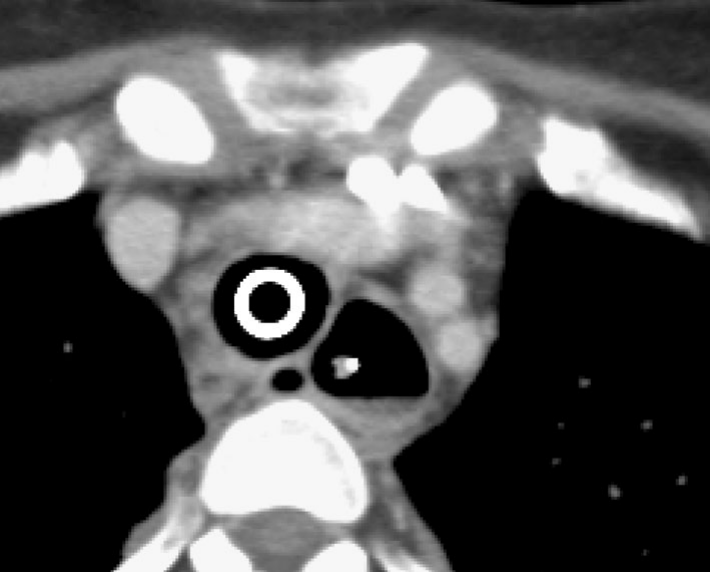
A follow-up computed tomography (CT) scan performed on the 15th day from the onset demonstrating the improvement in the diffuse esophageal wall thickening
